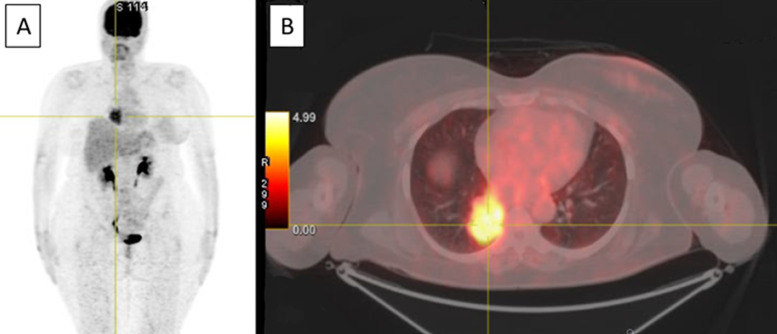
18F-fluorodeoxyglucose (18F-FDG) positron emission tomography-computed tomography (PET-CT) findings; A) full-body maximum intensity projection (MIP) image showing focal hypermetabolic uptake in the right lung; B) axial fused PET-CT image demonstrating hypermetabolic activity corresponding to the right mediobasal pulmonary lesion
